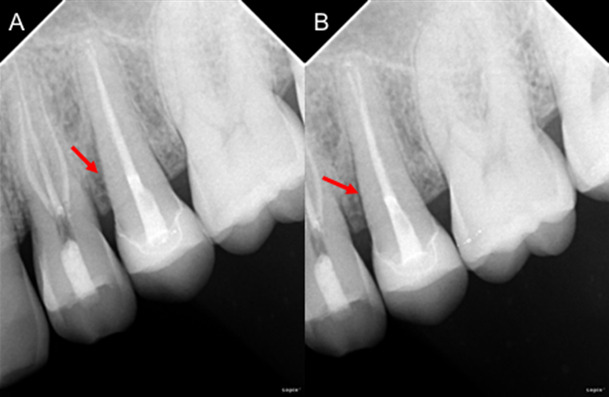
Caso 3. Radiografías periapicales postoperatorias que con variación de la angulacion horizontal que permiten evidenciar limitadamente el defecto óseo mesial al diente 2.5.
